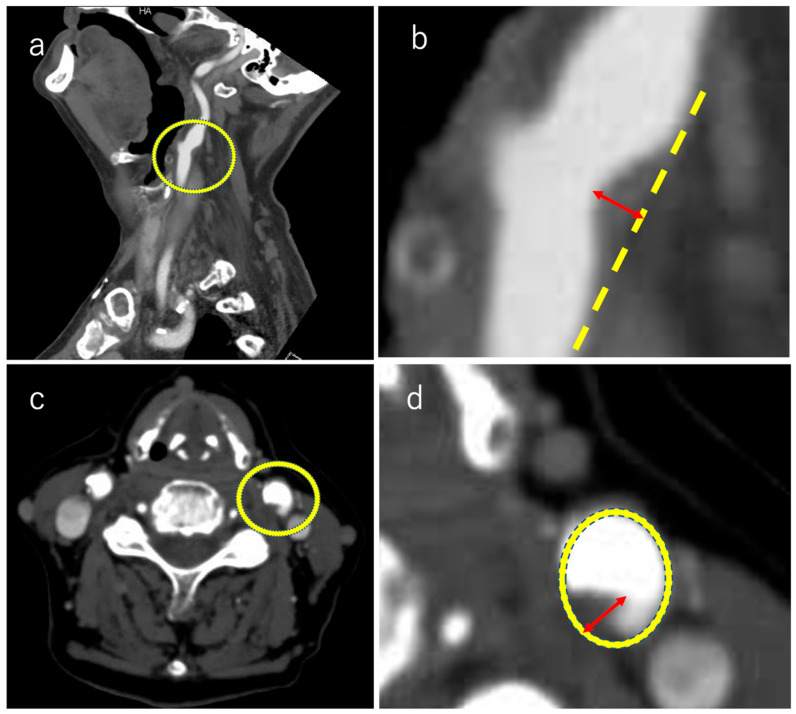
Measuring plaque size using computed tomography angiography. (a) Sagittal and (b) enlarged images of the plaque area. A line perpendicular to the vessel wall is drawn, and its maximum diameter is measured. Similarly, the axial view (c) and enlarged image of the plaque area is shown (d). A line perpendicular to the vessel wall is drawn to measure its maximum diameter.
